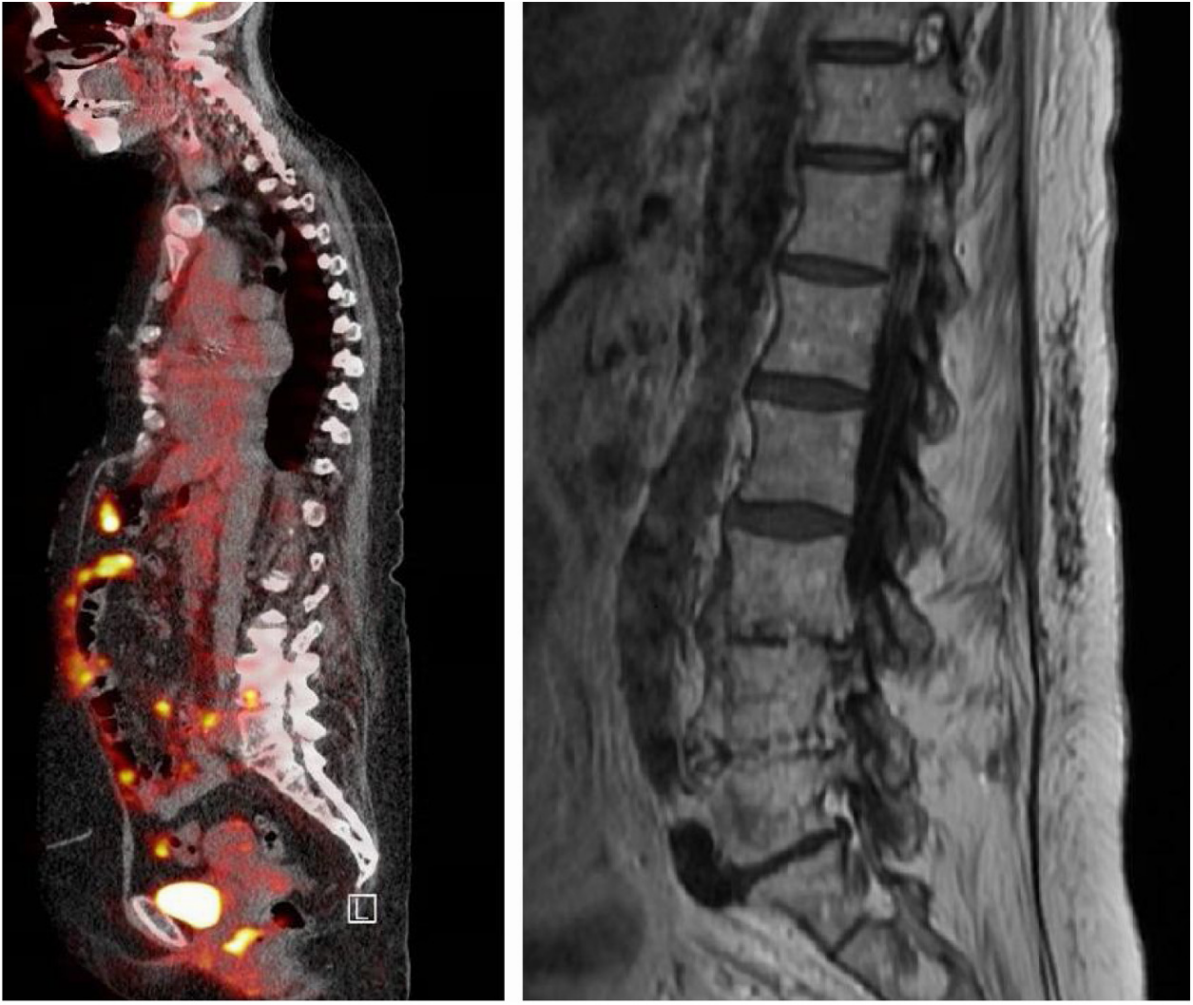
PET-CT scan (left panel) and MRI scan (right panel) of patient B revealing increased FDG-uptake at lumbar discus L4-L5, indicative for spondylodiscitis.
